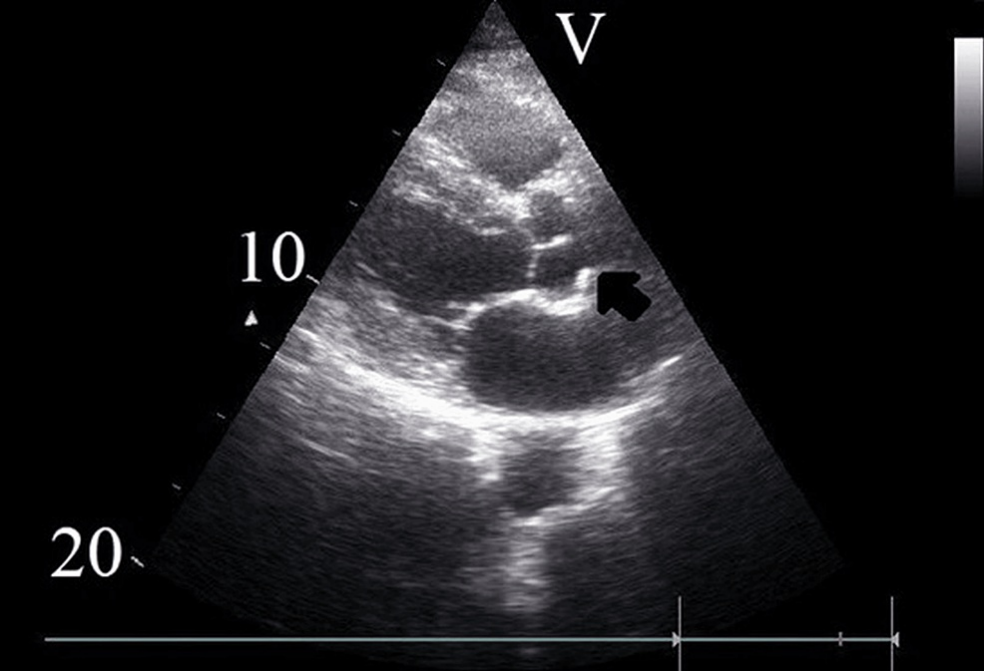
Evaluation of calcification in the aortic root by echocardiography in patients with knee osteoarthritis.
